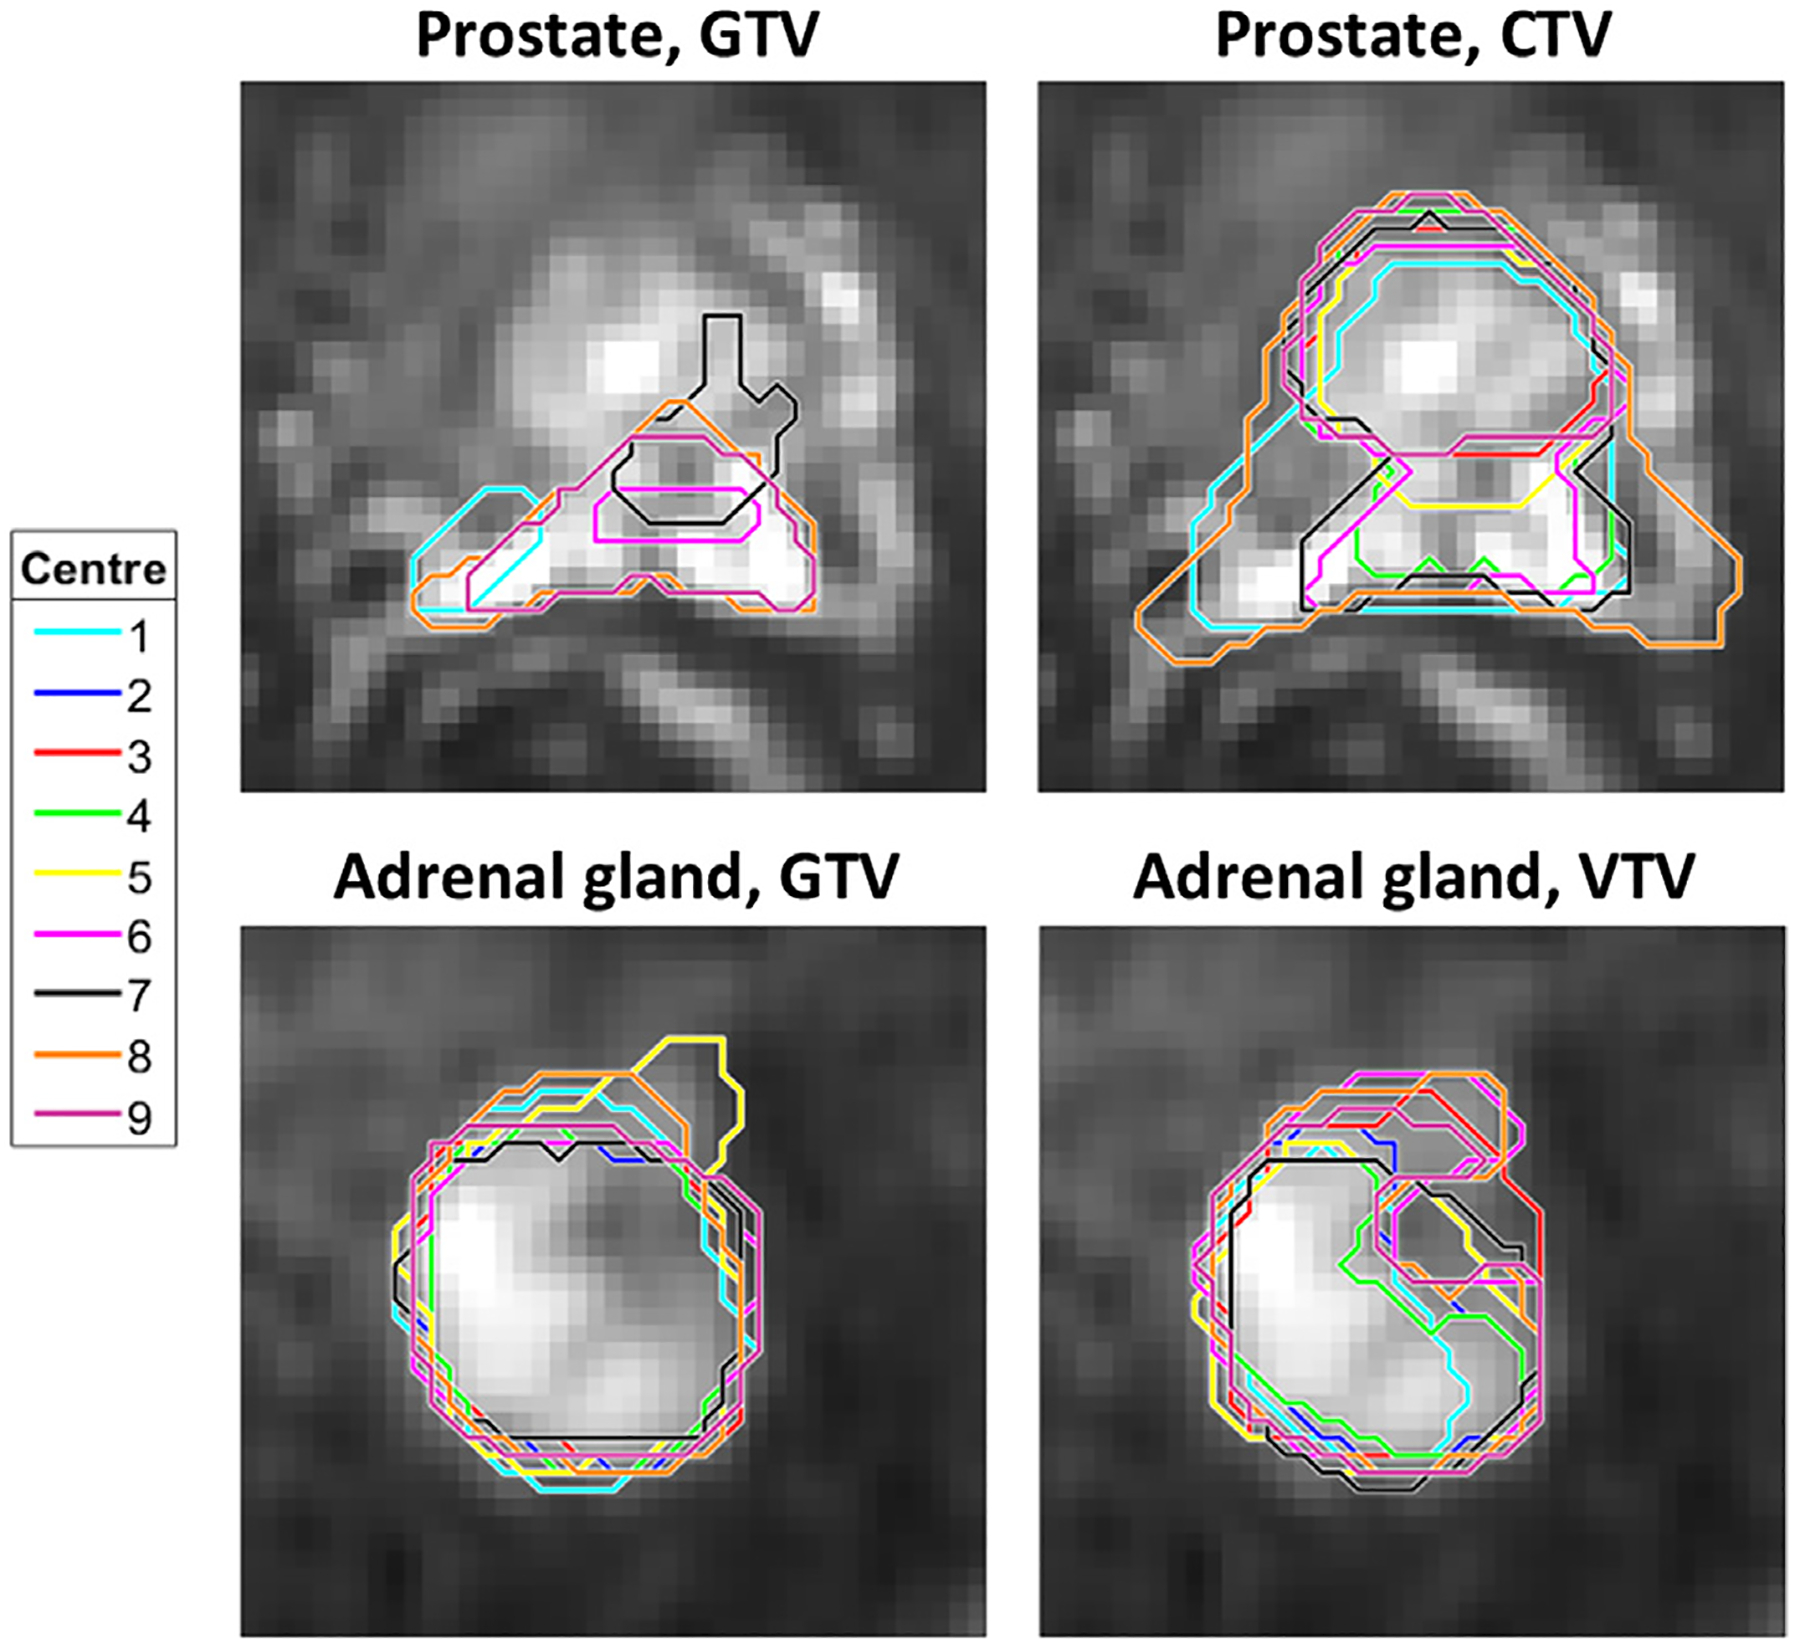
Examples of delineations. Delineations made by the nine participating centres for prostate and adrenal gland, shown on b = 500 /mm^2^ DWI images, cropped to an area of 7.7 × 7.7 cm^2^ (prostate) and 4.9 × 4.9 cm^2^ (adrenal gland) around the tumour. For the prostate, not all delineated contours included the shown slice, thus, only five contours are visible.
